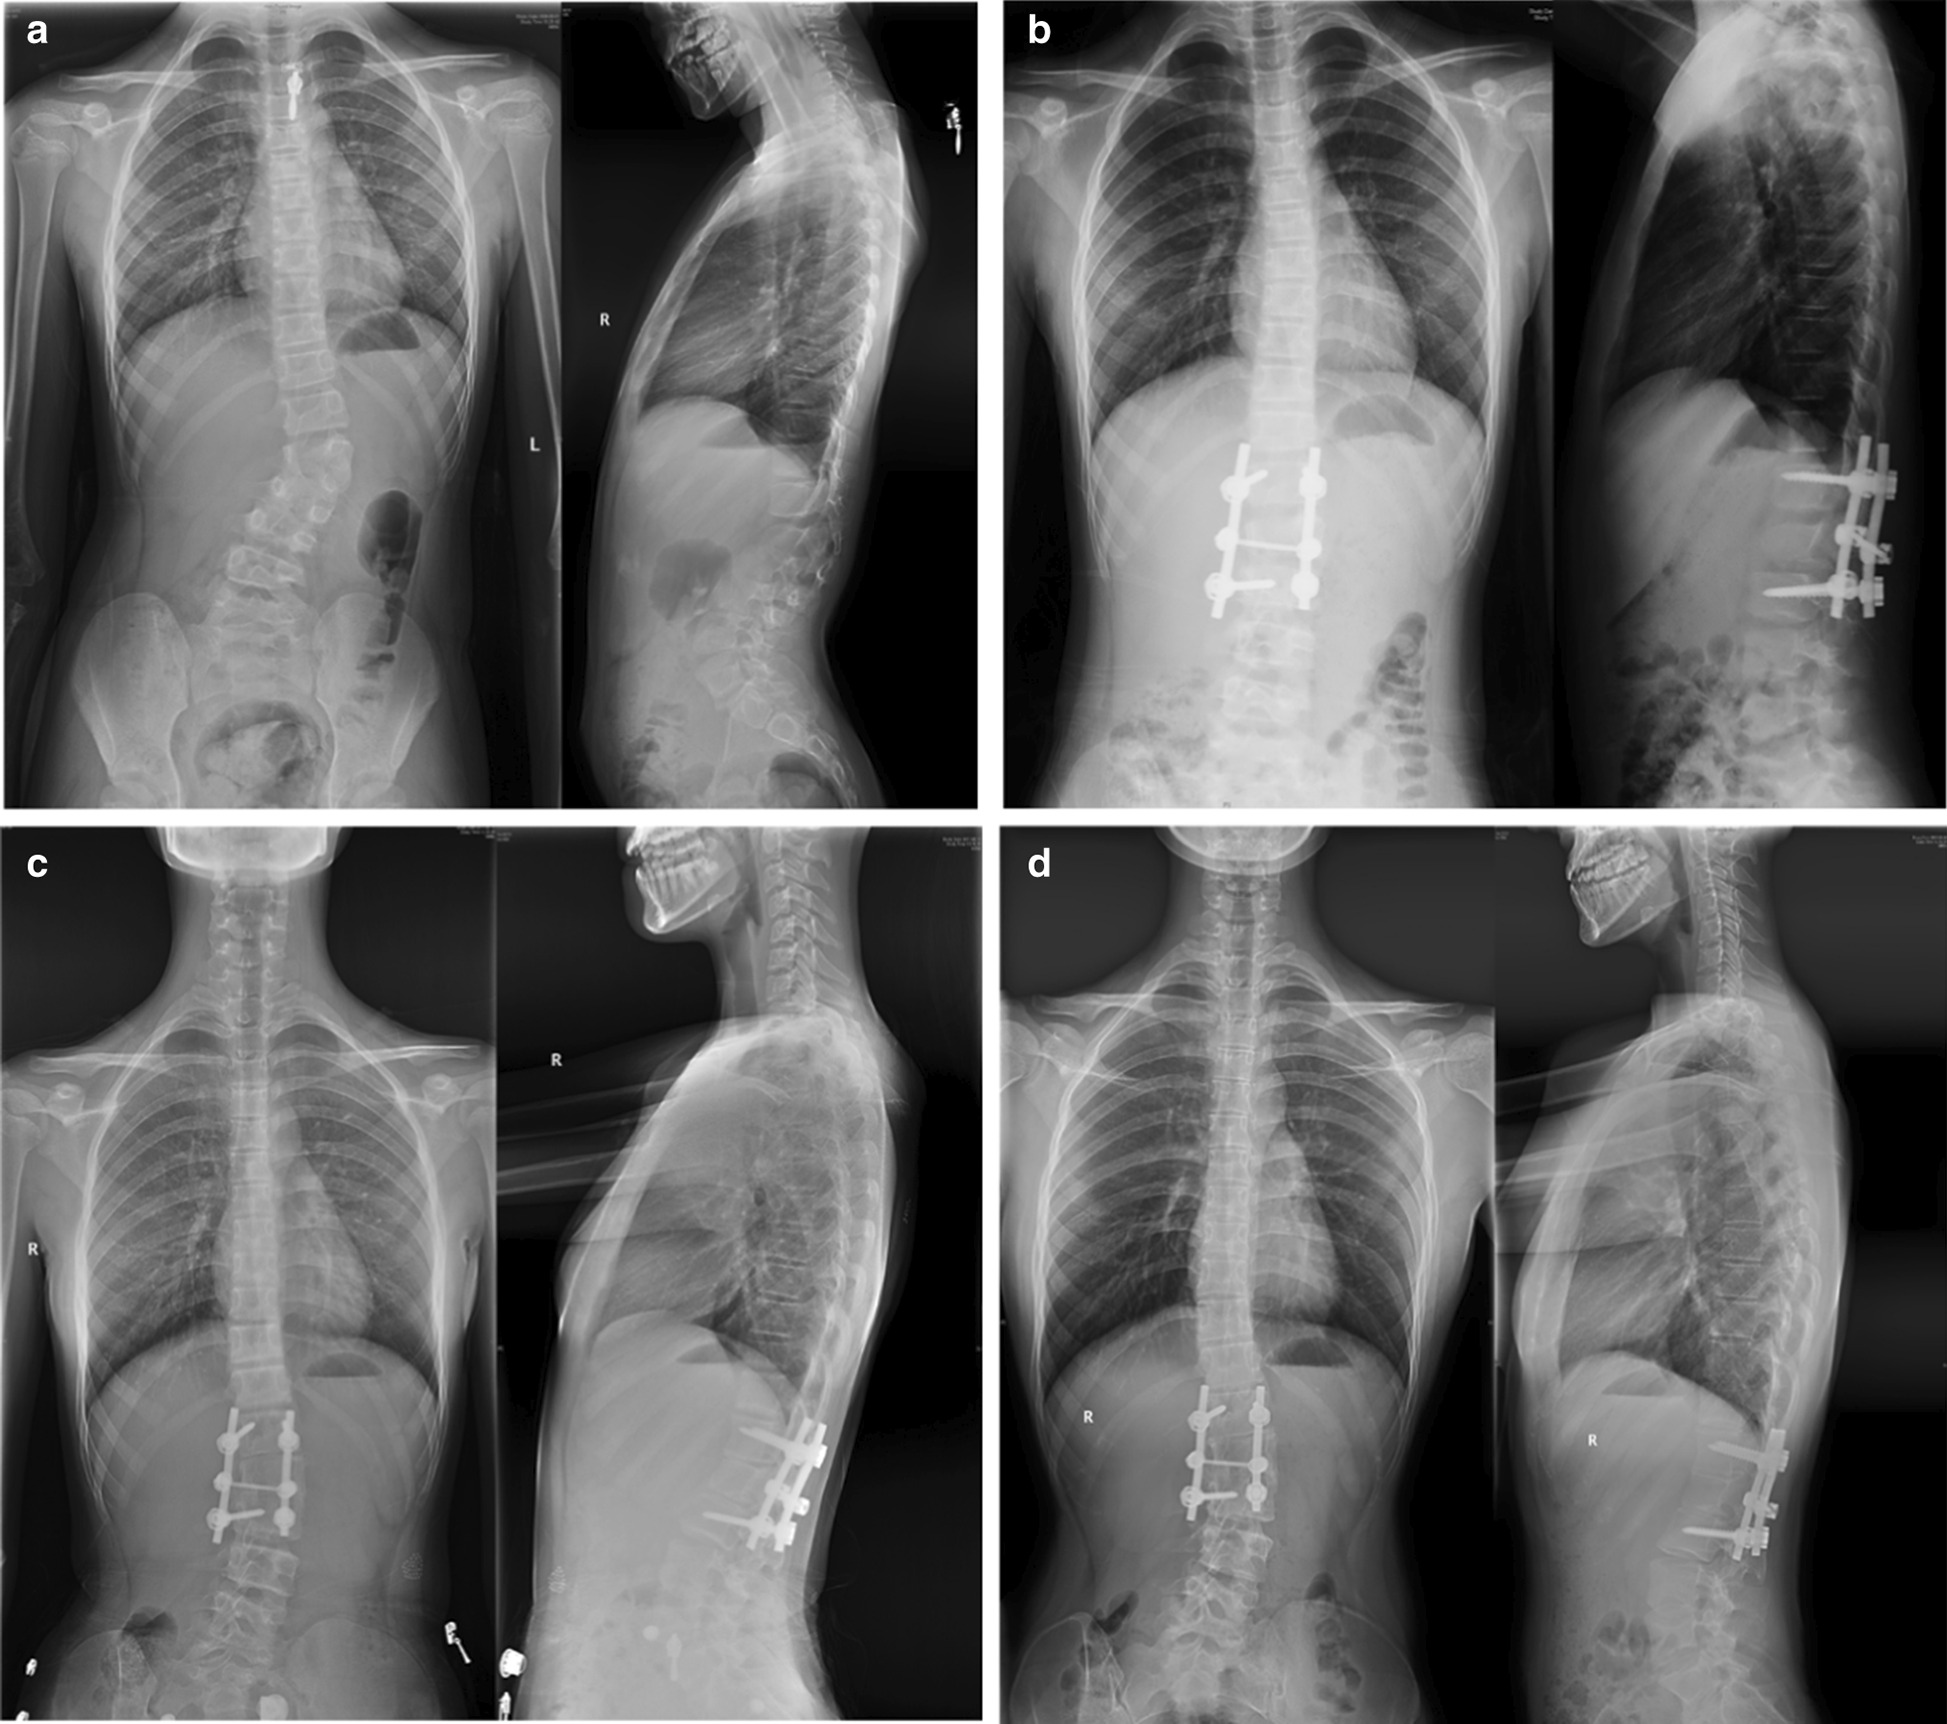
Radiograph of a patient with congenital scoliosis. A hemivertebra is located at L2 (second lumbar vertebra). The standard standing long cassette anterior–posterior and lateral radiographs were performed preoperatively (a), postoperatively (b), at 2-year follow-up (c), and at the 10-year follow-up visit (July 8, 2019) (d). The patient demonstrated the adding-on phenomenon at 2-year follow-up
